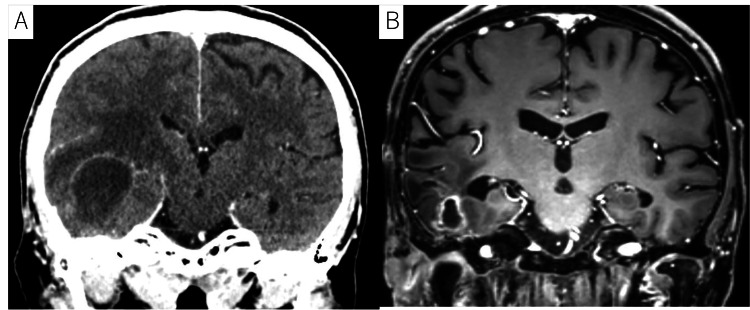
Images of the brain abscess in case 2 A: CT scan demonstrating a ring-enhancing lesion with a low-density area in the right temporal lobe, consistent with a temporal lobe abscess, measuring a maximum of 36 mm. The brain abscess developed two months after RT. B: The brain abscess persisted despite drainage and antibiotic treatment.
